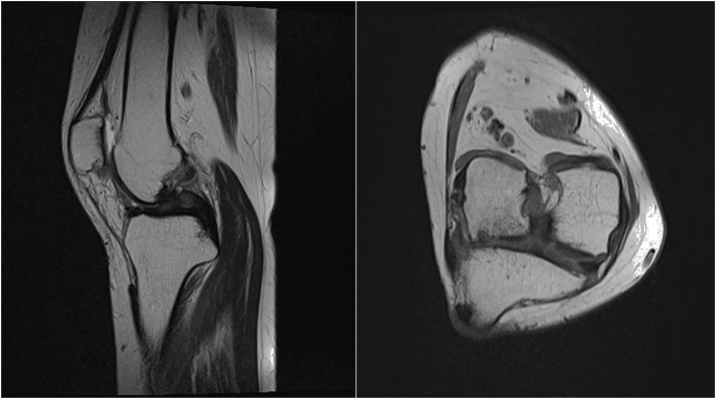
There was no identifiable structure with the recognizable features of the anterior cruciate ligament. A : T2 weighted sagittal MR image. B: T2 weighted axial MR image.
